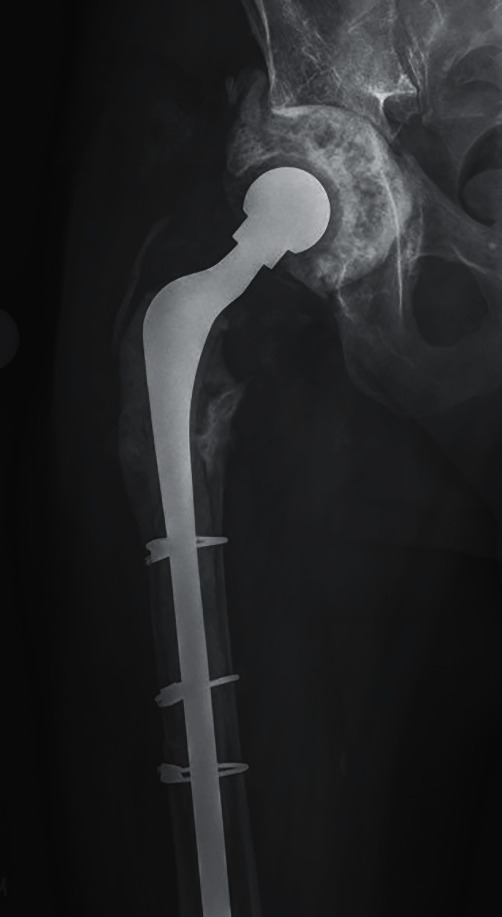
Anteroposterior X-ray of the right hip with antibiotic spacers.
